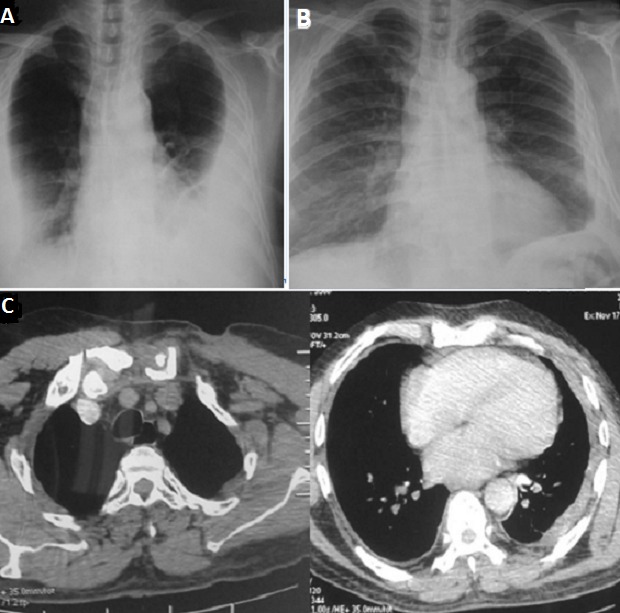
(A) radiographie du thorax à l’admission: opacité bilatérale de type pleural; (B) radiographie du thorax à la fin du traitement: régression de l’opacité bilatérale; (C) tomodensitométrie thoracique: régression de la pleurésie et de la masse pariétale
